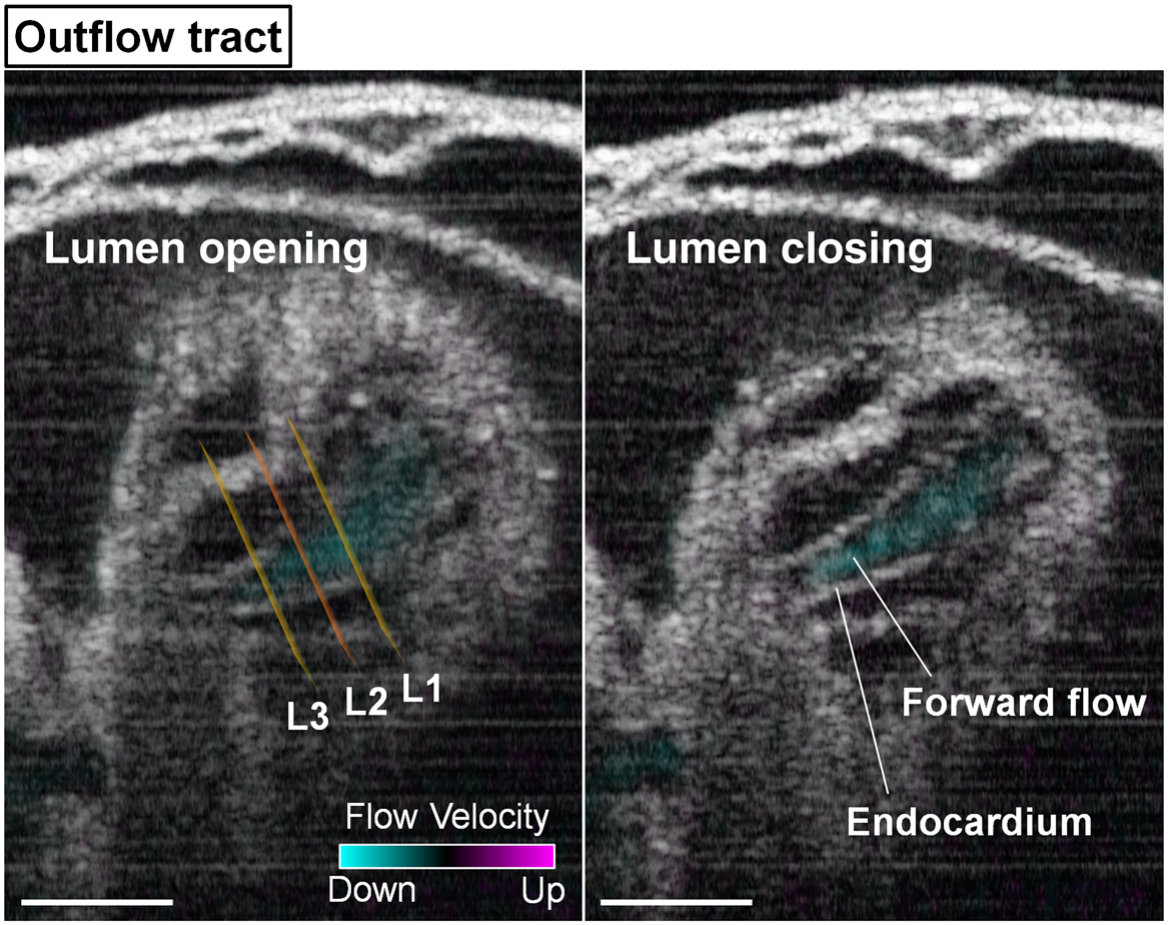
Structural and Doppler 4D-OCT imaging of the outflow tract in E9.25 embryonic heart (Video 4). The positions of the measurement planes L1, L2, and L3 are shown in the left panel. Scale bars are 100  μm (Video 4, mov, 8.64 MB [URL: https://doi.org/10.1117/1.JBO.25.8.086001.4]).
